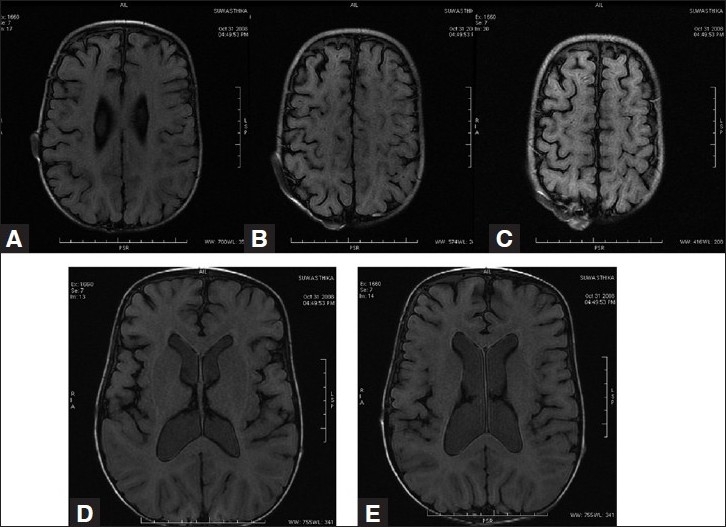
(A–C) T1-weighted images show the hypointense scalp vein with bony defect and venous sac. (D, E) The dilated ventricular system
